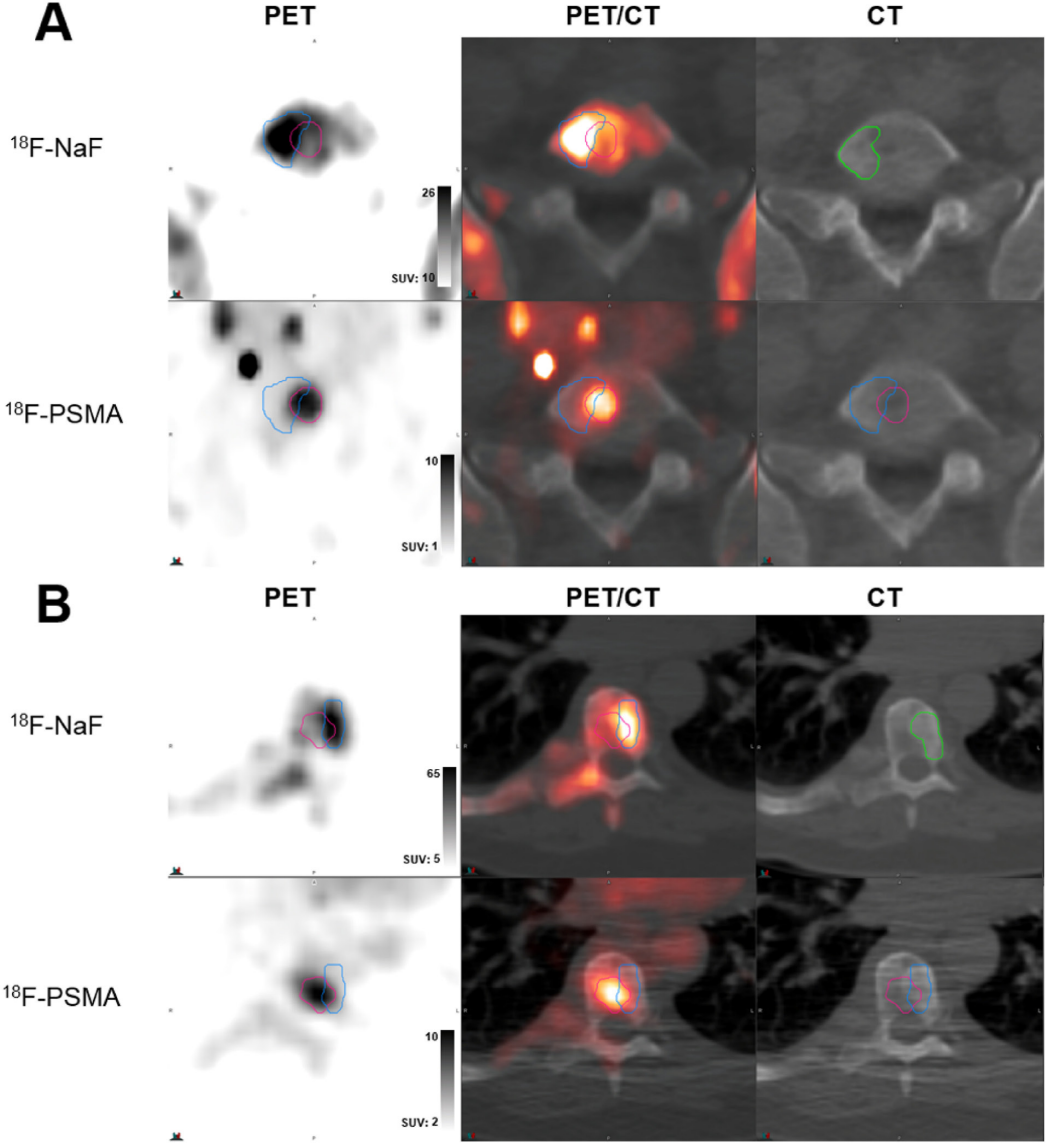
Examples of low volume and spatial uptake concordance (A) Lesion in L5 spine of castrate-resistant patient (serum PSA 11.79 ng/ml) demonstrating poor 62.2% volumetric overlap between ^18^F-NaF uptake (top row, blue contour) and ^18^F-PSMA (DCFPyL) (bottom row, red contour) uptake, with marked mismatching patterns of high uptake within contours. (B) Lesion in T12 spine of castrate-resistant patient (serum PSA 4379 ng/ml) demonstrating high 30.9% volumetric overlap between ^18^F-NaF uptake (top row, blue contour) and ^18^F-PSMA (DCFBC) (bottom row, red contour) uptake, with substantial mismatch in uptake patterns. In both (A) and (B), top row demonstrates PET uptake, PET/CT overlay, and low-dose CT from ^18^F-NaF scan and bottom row demonstrates PET uptake, PET/CT overlay, and low-dose CT from PSMA scans with registered contour overlay. CT contours shown in green.
